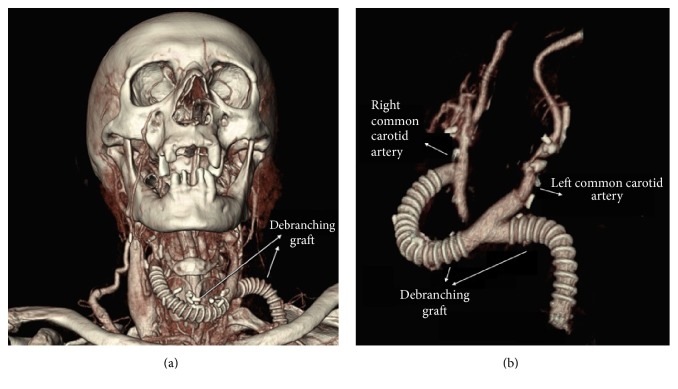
(a) Postoperative computed tomography (CT) angiography view of the debranching graft. (b) Debranching graft's proximal anastomosis was performed end to side to right common carotid artery; graft's carotid leg was anastomosed to left common carotid artery end to end; graft's left subclavian artery leg was anastomosed to the left subclavian artery end to side; and left subclavian artery was simply ligated proximally.
